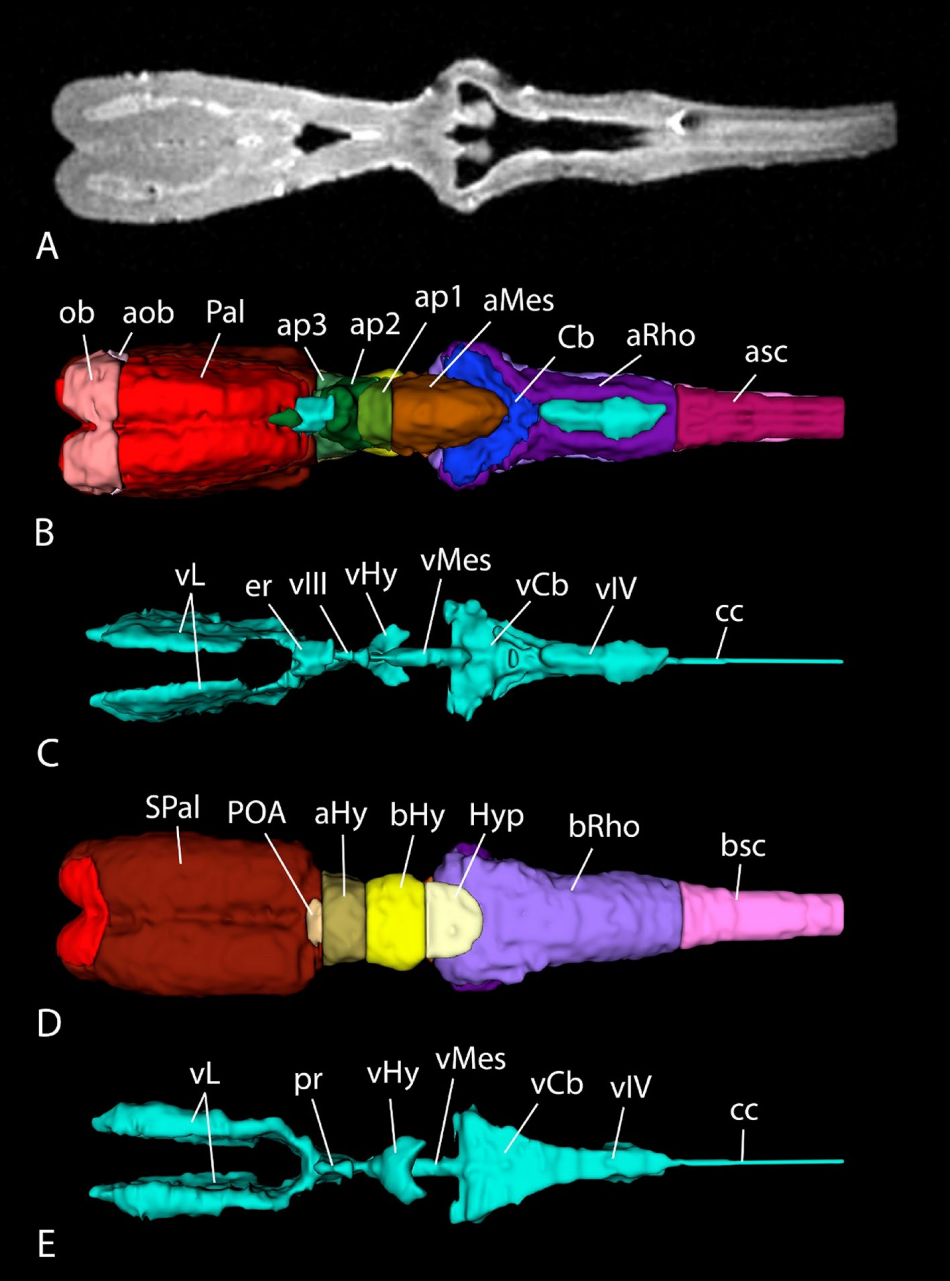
Horizontal MR image (A) and 3D reconstructions of the brain (B,D) and the ventricular system (C,E) of *Protopterus annectens* in dorsal (B,C) and ventral (D,E) views. *aHy* alar hypothalamus, *aMes* alar mesencephalon, *aob* accessory olfactory bulb, *ap1* alar part of p1, *ap2* alar part of p2, *ap3* alar part of p3, *aRho* alar rhombencephalon, *asc* alar spinal cord, *bHy* basal hypothalamus, *bRho* basal rhombencephalon, *bsc* basal spinal cord, *Cb* cerebellum, *cc* central canal, *er* epithalamic recess, *Hyp* hypophysis, *ob* olfactory bulb, *Pal* pallium, *POA* preoptic area, *pr* preoptic recess, *SPal* subpallium, *vCb* cerebellar ventricle, *vHy* hypothalamic ventricle, *vIII* third ventricle, *vIV* fourth ventricle, *vL* lateral ventricles, *vMes* mesencephalic ventricle.
